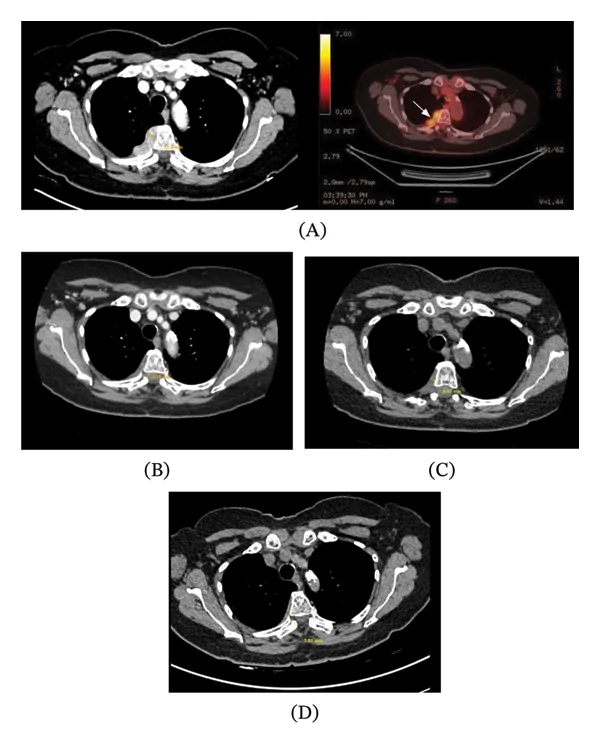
Imaging (axial view) of the chest showing the right‐sided paraspinal mass. (A) CT scan (left) and PET scan (right) at the time of diagnosis. The thickness of the mass measured 12.3 mm, and the corresponding PET scan showed an SUV of 6.5. (B) CT scan 9 months after starting prednisone, showing shrinkage of the mass to 6.15 mm. (C) CT scan 4 months after starting rituximab, showing thickness of the mass to be stable at 6.07 mm. (D) CT scan 3 years after initiation of rituximab infusions every 6 months, showing a reduction in the mass size to 3.82 mm.
